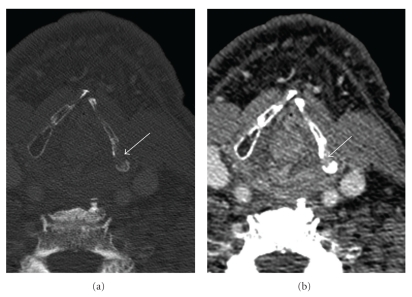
Chondronecrosis of the thyroid cartilage: (a) Axial contrast-enhanced CT of the thyroid cartilage (bone window) shows destruction of the left side of the cartilage (arrow). (b) Axial contrast-enhanced CT (soft tissue window) shows no evidence of an adjacent enhancing soft tissue mass (arrow).
